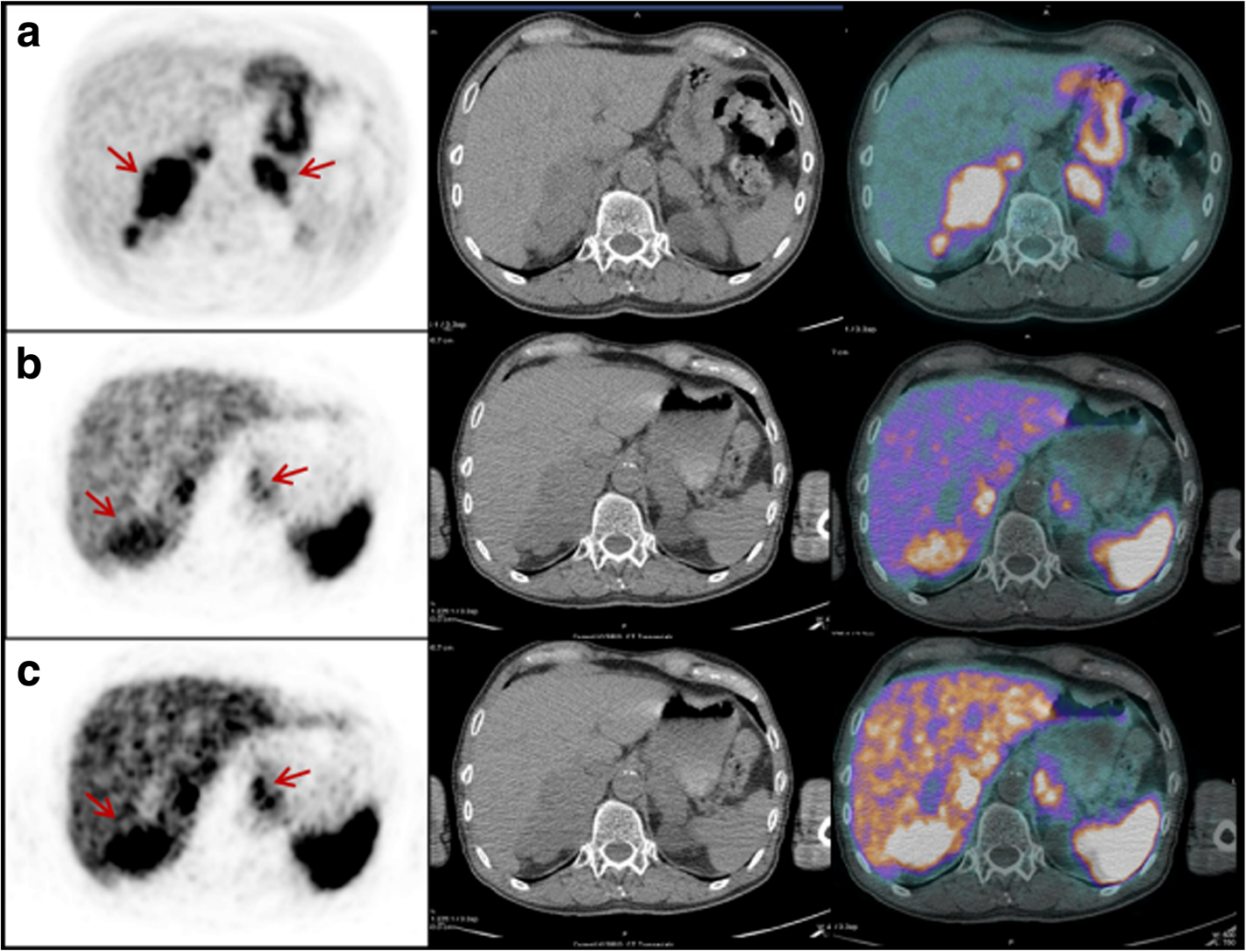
PET/CT images performed for re-staging in patient #14. Transaxial ^18^F-FDG (a) and ^68^Ga-somatostatin analogs (b, c) at different intensity levels. PET/CT images showed abnormal tracer uptake in both adrenal metastatic lesions (red arrows). Both adrenals were enlarged (right > left). The pattern of uptake was inhomogeneous with both ^18^F-FDG and ^68^Ga-somatostatin analogs and, in the whole, a “reverse” and complementary distribution of uptake was evident with the two tracers, with clearly parts of the tumor that take up one or the other tracer (e.g., the “hottest” part of the right adrenal at ^18^F-FDG appears substantially “cold” at ^68^Ga-SRI)
